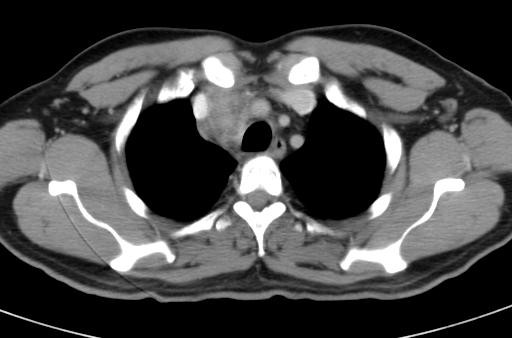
Computed tomography chest scan showing anterior mediastinal mass in the right paratracheal region.
